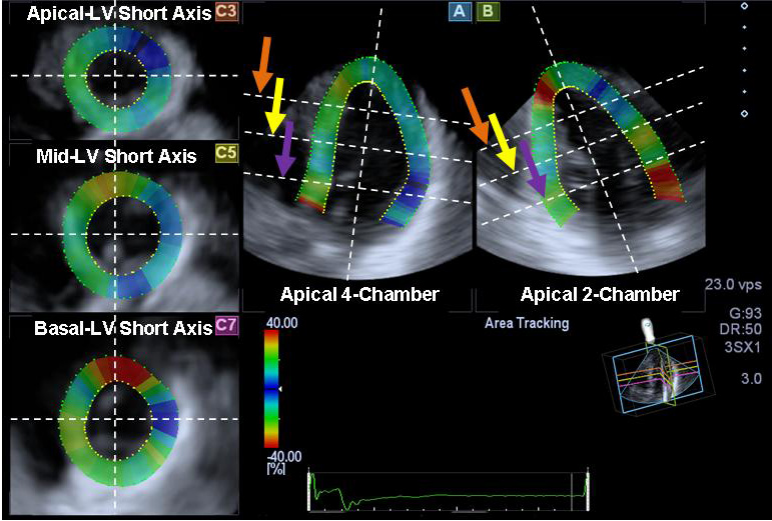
Example of images generated from a pyramidal three-dimensional dataset: (A) apical 4-chamber view, (B) 2-chamber view, (C3) apical left ventricular (LV) short-axis view (orange arrow), (C5) mid-LV short-axis view (yellow arrow), and (C7) basal LV short-axis view (purple arrow).
